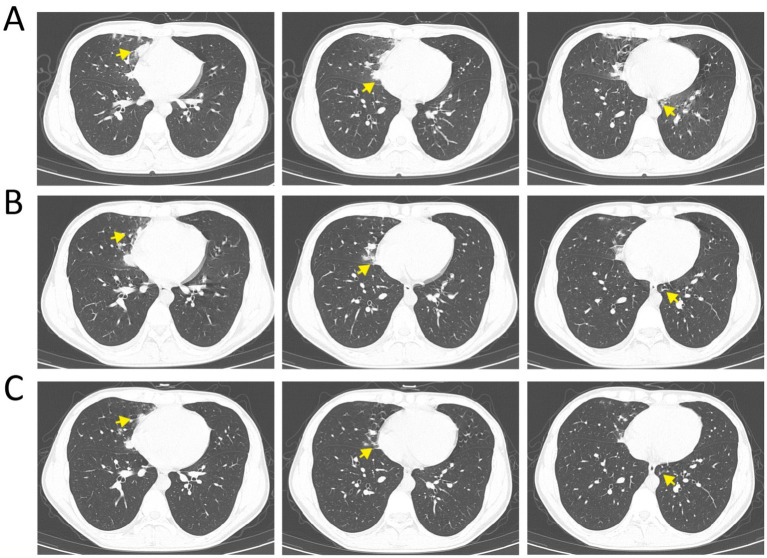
Panels (A–C) correspond to chest CT images obtained on hospital days 1, 3, and 7, respectively. Yellow arrows indicate the affected lesions.
